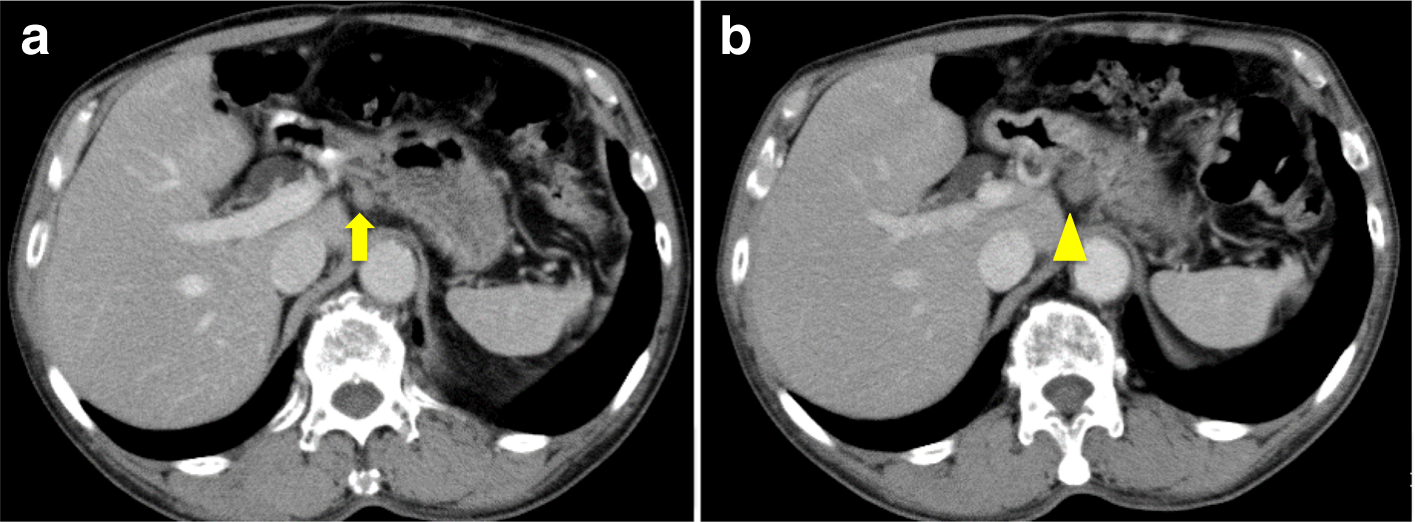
An abdominal contrast-enhanced computed tomography a before endoscopic submucosal dissection (ESD) and b 1.5 months after ESD. a Abdominal contrast-enhanced computed tomography shows a nodule lesion with slight contrast near the remnant stomach (arrow). b The nodule lesion increased in size over 3 months (arrow head)
